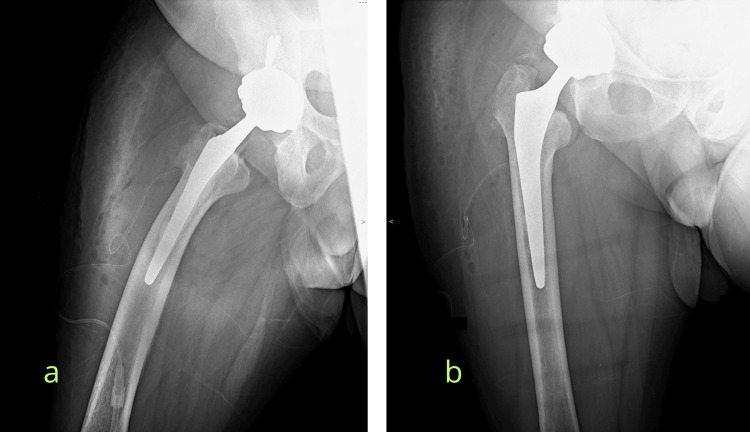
Final radiographs after the second revision. Lateral (a) and anteroposterior (b) radiographs of the right hip following the one-stage revision total hip arthroplasty.
